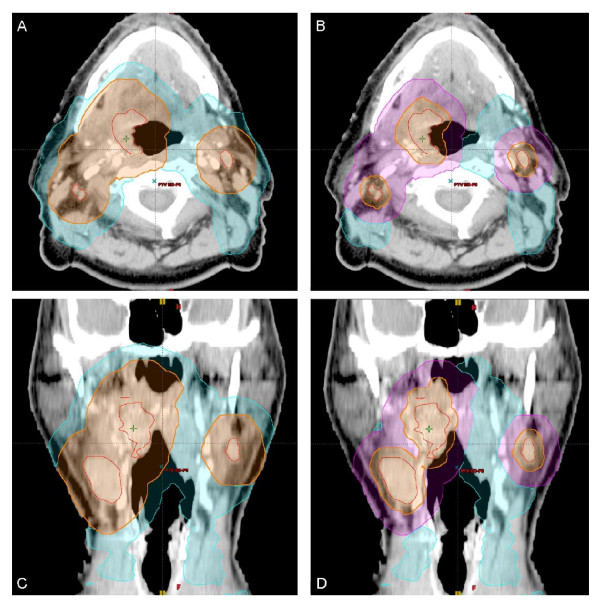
Representative patient demonstrating variation in planning target volumes (PTV) for two dose (RTOG) and three dose (3Dose) plans. Axial (A-B) and coronal (C-D) computed tomography images shown. The gross tumor volume (GTV, red line) is identical for each plan. The PTV-high dose (PTV-HD, orange) and PTV-elective dose (PTV-ED, cyan) are presented for RTOG in A and C, respectively. These volumes plus PTV-intermediate dose (PTV-ID, magenta) are displayed for 3Dose in B and D.
